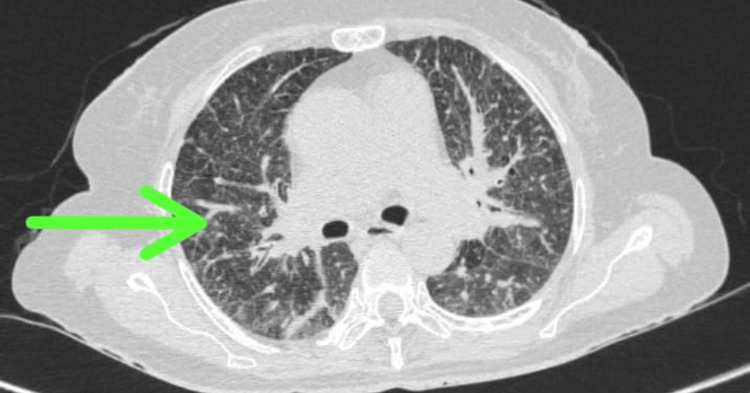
High-Resolution CT of the Chest
